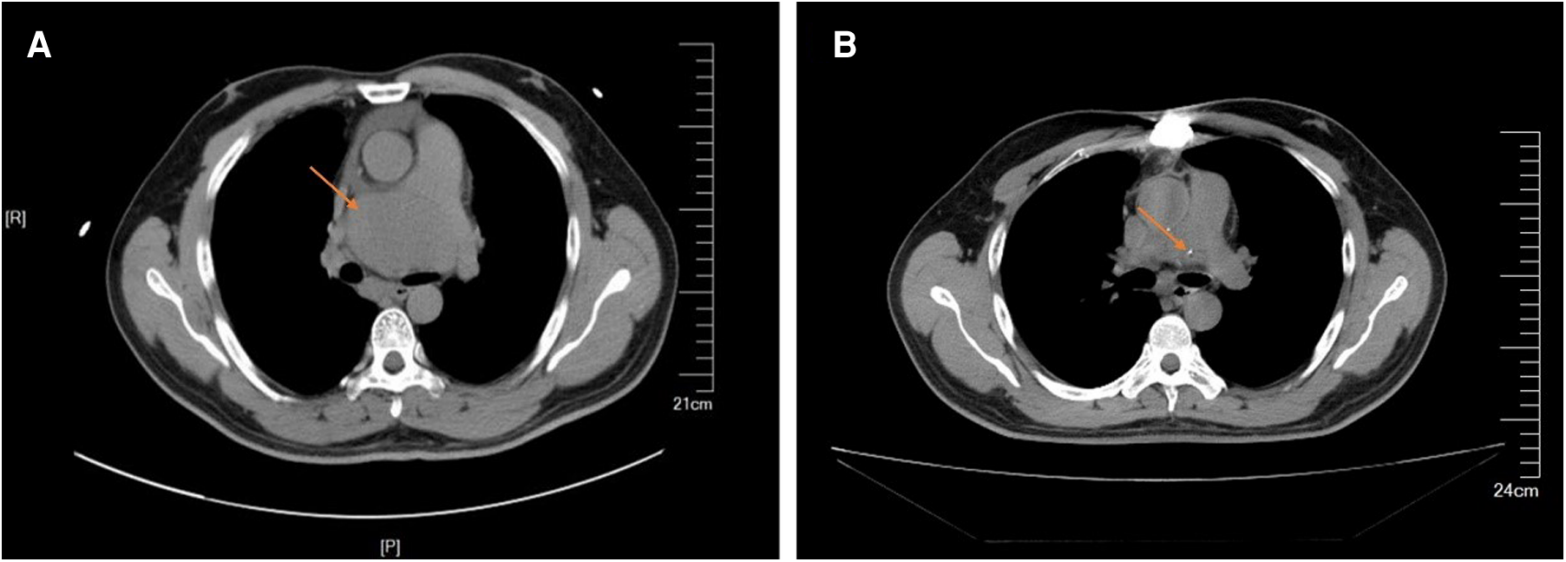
(A) Preparative computed tomography scan showed that the mass close to the main pulmonary artery bifurcation and extended along the right pulmonary artery. (B) Postoperative computed tomography scan showed no residual and no recurrence of the tumor.
